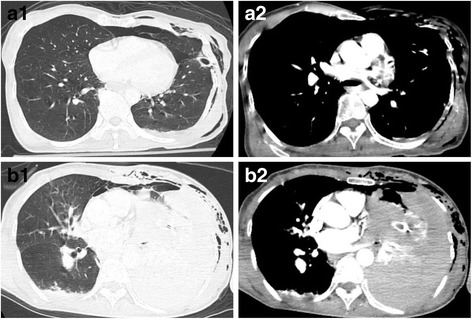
Comparison of the chest computed tomography images at initial arrival (a1 and a2) and post-embolization (b1 and b2). Pneumothorax and subcutaneous emphysema were recognized at the initial diagnosis by computed tomography and, therefore, a chest drain was inserted. Pulmonary contusion was extremely minor and active bleeding such as the intercostal artery injury was not captured (a1 and a2). A large hemothorax appeared on reexamination by computed tomography after transcatheter arterial embolization (b1 and b2). Similar active bleeding was not observed at the initial diagnosis computed tomography. A large hemothorax of 2500 ml was observed in thoracotomy hemostasis. However, active bleeding requiring hemostasis treatment was not recognized. The inserted chest drain was occluded by blood clots
